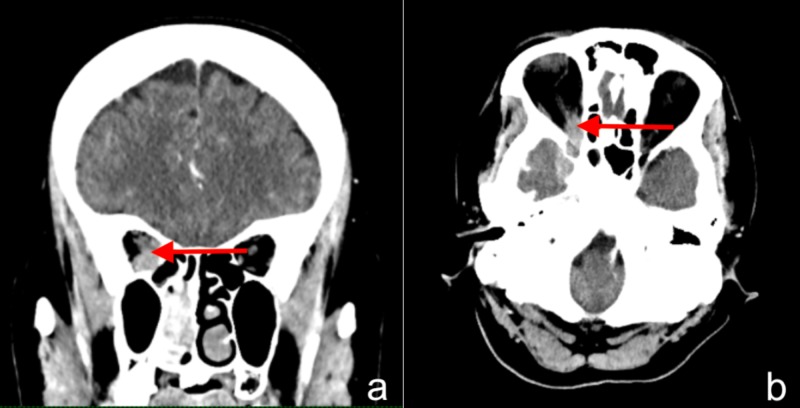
CT scan of orbit showing the mass encasing the right intra-orbital optic nerve at the orbital apex (red arrow) in the coronal view (a) and axial view (b).
